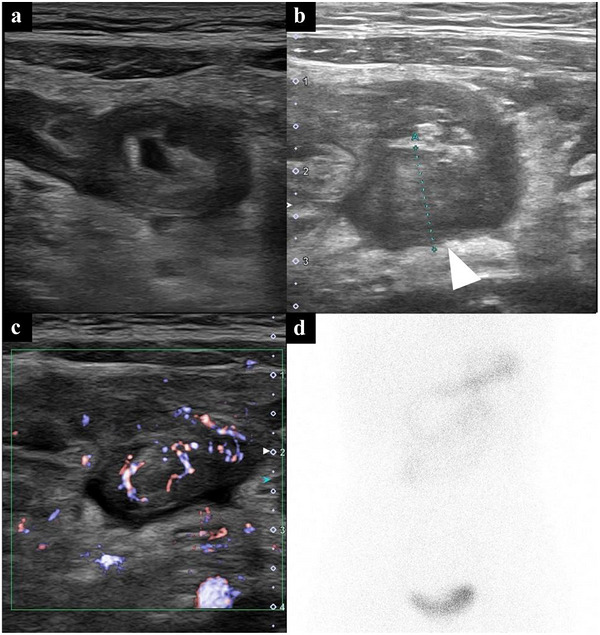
Ultrasonographic and Technetium‐99^m^ pertechnetate scintigraphy findings in Case 2. (a) Localized thickening of the ileal wall was observed. (b) A blind‐ending tubular structure (arrowhead) was visualized, consistent with a diverticular lesion. (c) Advanced dynamic flow demonstrated increased intralesional blood‐flow signals, and the surrounding mesenteric fat showed enhanced echogenicity consistent with inflammation. (d) No definite abnormal uptake was detected on Technetium‐99^m^ pertechnetate scintigraphy.
